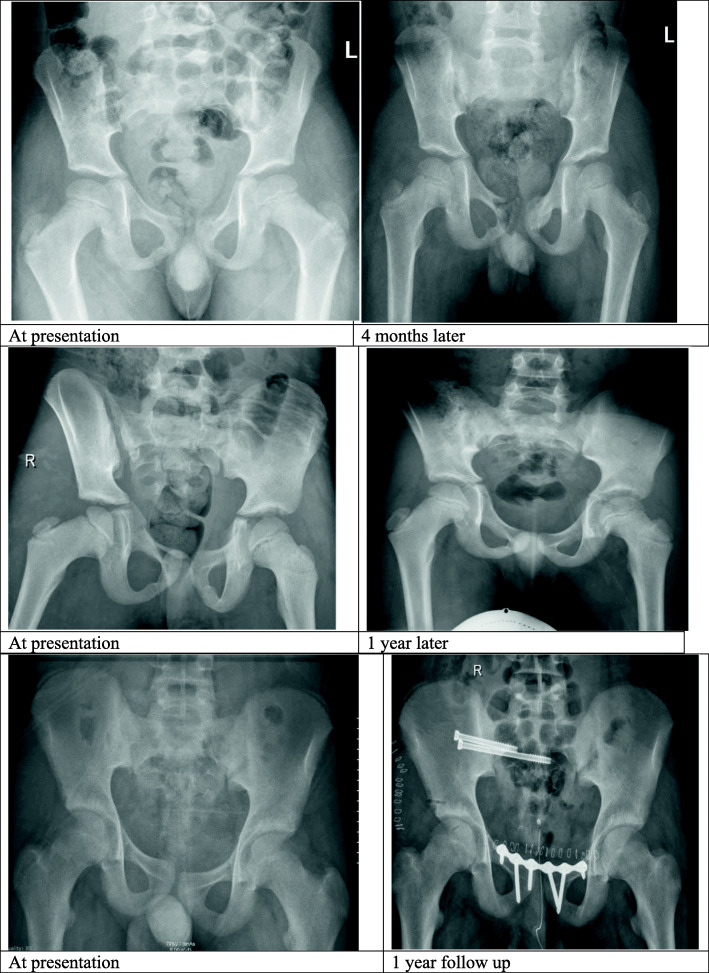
Hip X-ray before and after treatment of pediatric pelvic fractures: the upper panel shows Torode type III, treated non-operatively by non-weight bearing for 6 years old patient. The middle panel shows Torode type IV, treated by skeletal traction in 8 years old patient. The lower panel shows Torode type IV, treated by open reduction and internal fixation in 14 years old patient
